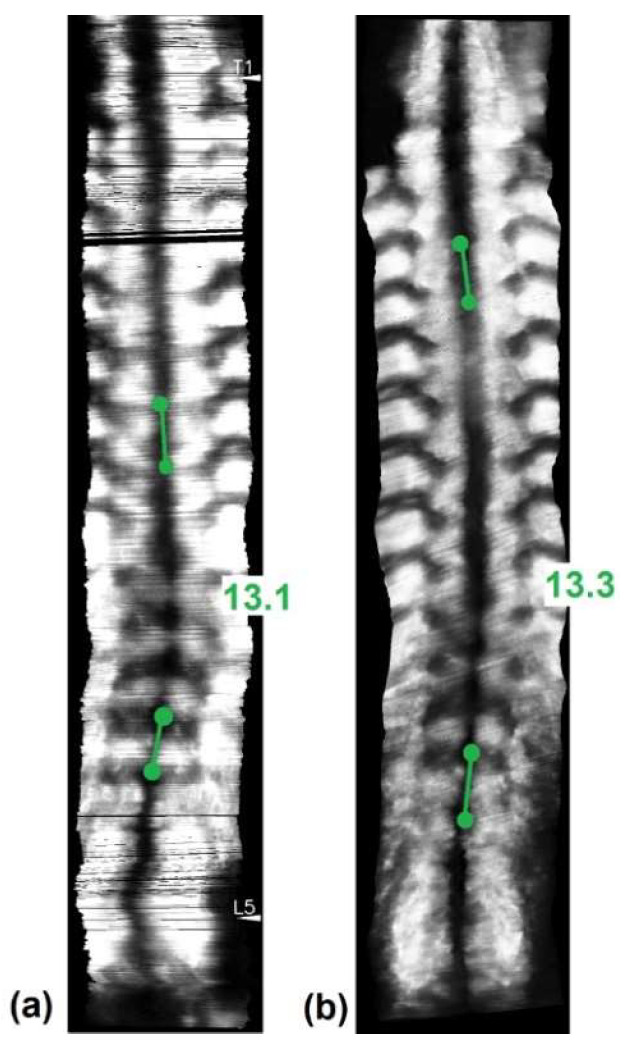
Coronal ultrasound images formed using the skin surface curve VPI method with SPA measurement on (a) Scolioscan Air and (b) Scolioscan.
